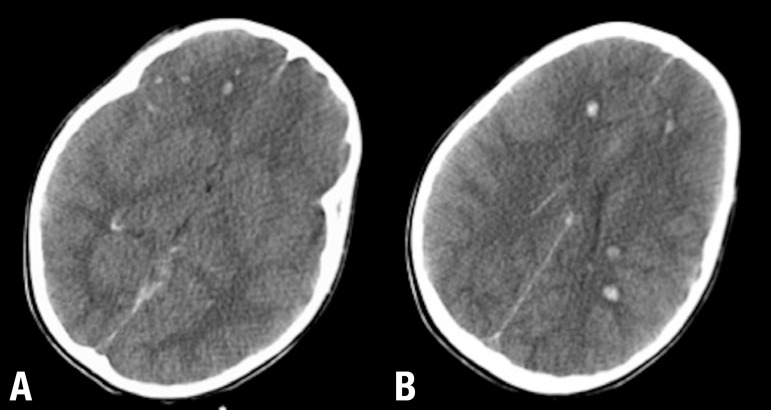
DAI in a patient with severe TBI. Axial non-contrast CT [A and B] show
